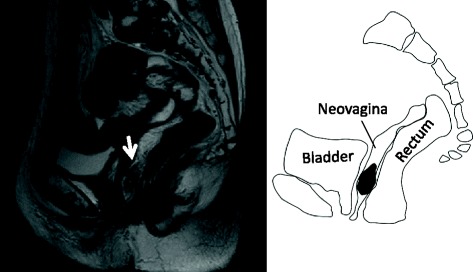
T2-weighted MR image. The neovagina existed between the bladder and rectum and extended from the vaginal opening to abdominal cavity. A 4.5-cm protruding lesion (white arrow) was detected along the posterior neovaginal wall
